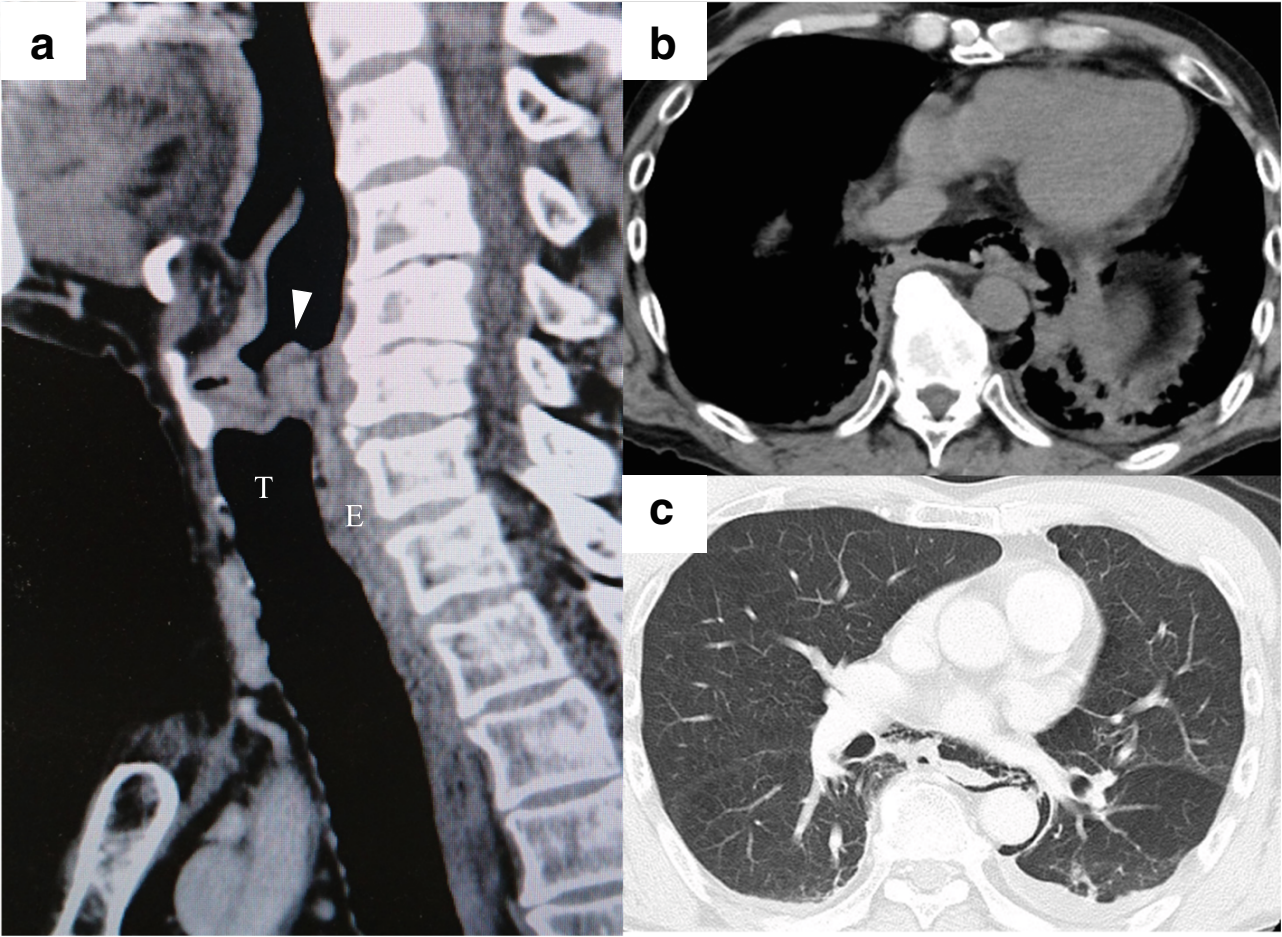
a Enhanced CT on sagittal section demonstrates stenosis caused by hypopharyngeal cancer involving the oropharnx (arrowhead, hypopharyngeal cancer). b, c Enhanced CT demonstrates extensive mediastinal emphysema, which ranged from the neck to the gastric cardia, and a small amount of left pleural effusion
